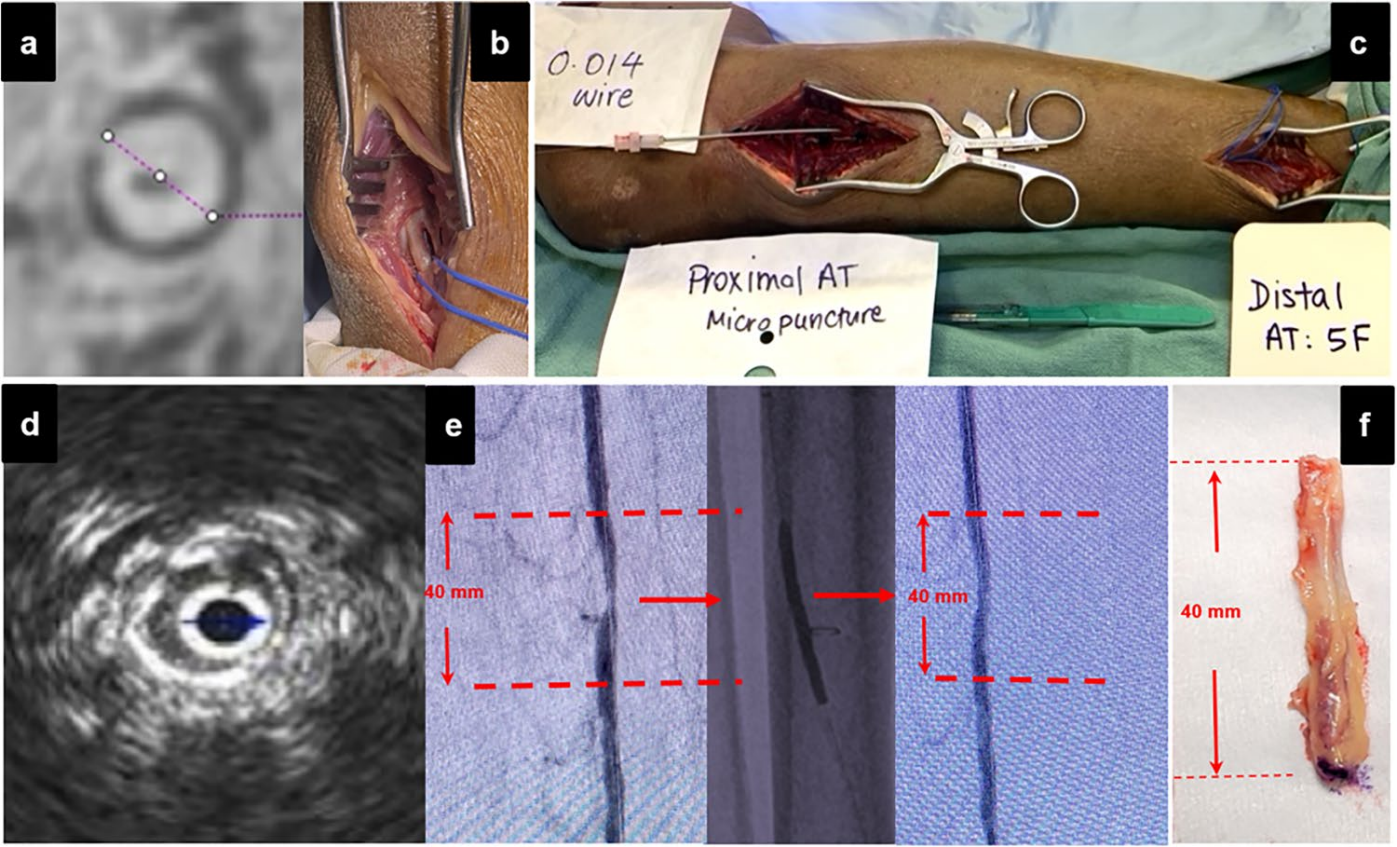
Experimental set up. a High-resolution MRI using ultrashort echo time sequences is used to characterize lesions at high resolution with the following parameters: 7 T: 28-channel clinical knee coil. UTE (FOV: 150 mm, TR 10 ms, TE 0.07 ms, flip angle 4). SSFP (FOV 160 mm, TR 12.57 ms, TE 6 ms, flip angle 25). The total acquisition time was 20 min (UTE, ultrashort echo time; SSFP, steady state free precession; FOV, field of view; TR, repetition time; TE, echo time). b The distal vessel was surgically exposed for arterial access under direct vision. c Amputated limb showing through and through 0.014″ wire access. d Intravascular ultrasound demonstrating concentric calcium in target lesion prior to intervention. e Angiography of the pre-intervention target lesion, angioplasty, and post-intervention completion angiogram. f Harvested target lesion for ex vivo analysis
